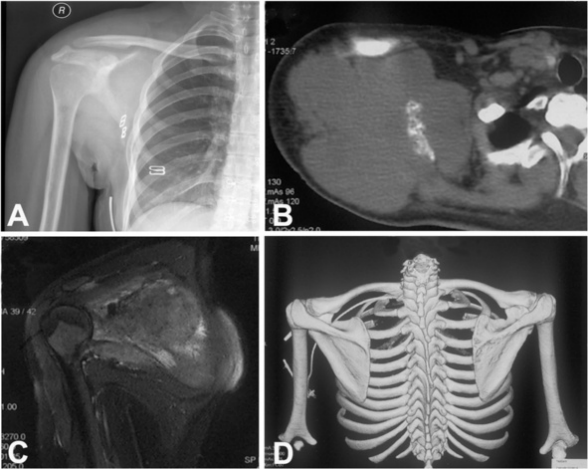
A 35-year-old woman with right scapular ES. a X-ray film showed osteolytic lesion with a bulky soft tissue shadow at right scapula. b, c CT and MRI showed destructed scapula and surrounding soft tissue mass. d 3-D CT reconstruction of affected scapula
